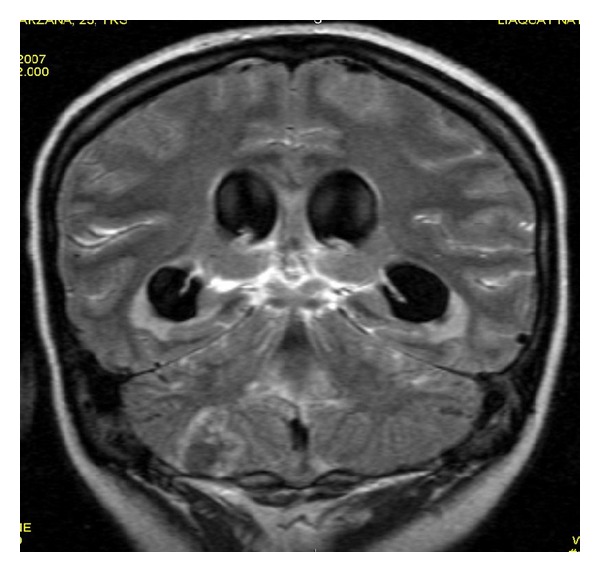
Postcontrast FLAIR image shows patchy areas of meningeal enhancement with dilated ventricles and irregular ring enhancing lesion representing postmeningitis abscess formation.
